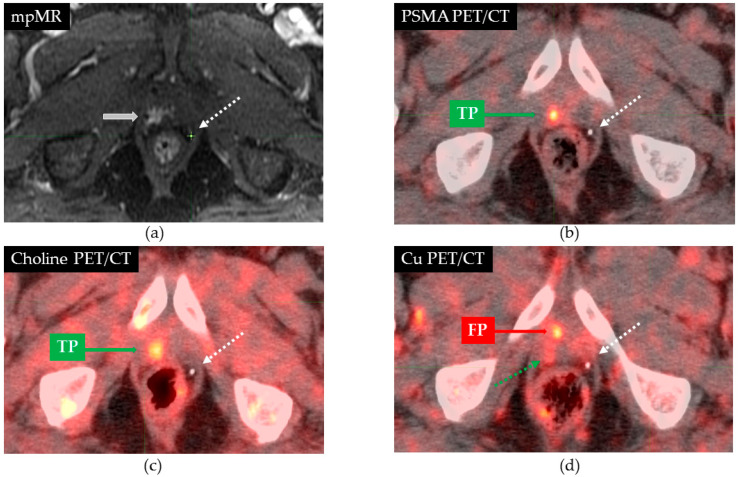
Axial slices showing the position of the nodule on the various imaging modalities: mpMR (a), PSMA/PET (b), CH/PET (c), and CU/PET (d). On multiparametric MR, the nodule is indicated by the thick white arrow. A focal lesion is shown on all PET/CT scans, while the dashed white arrow indicates the position of a calcification. The distances between the centroid on mpMR and PSMA PET/CT, Cu PET/CT, and choline PET/CT were 0.43, 1.21, and 0.47 cm, respectively. Therefore, lesions seen on both PSMA and choline PET/CT were considered true positives (TP), while the one on CU PET/CT was a false positive. Interestingly, on Cu PET/CT, a blurred mild uptake was detected in the area that would correspond to the TP lesion on mpMR (green dashed line).
